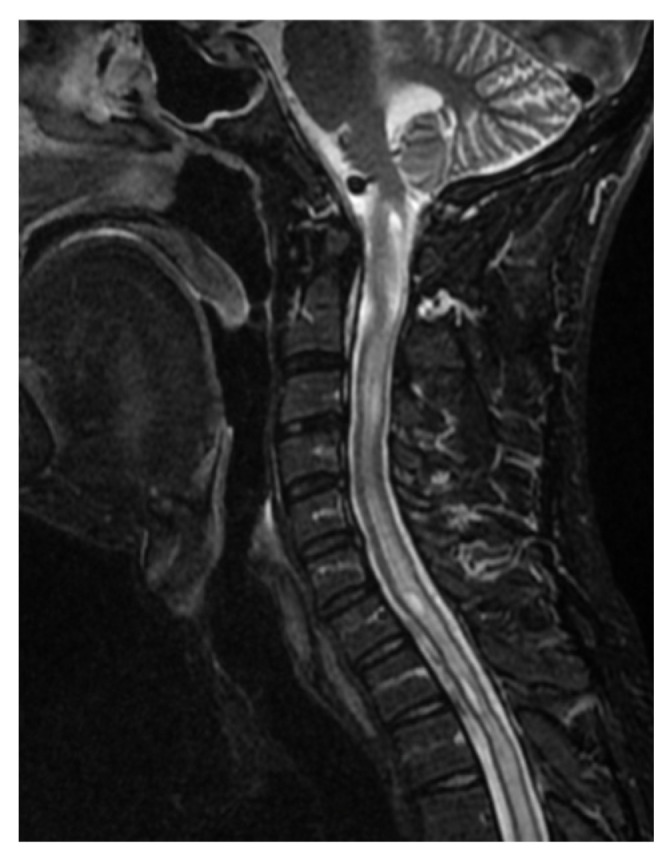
Significant progression of syringomyelia involving the entire spinal cord. Cervical spine. Single Sagittal Scan. MRI T2.
